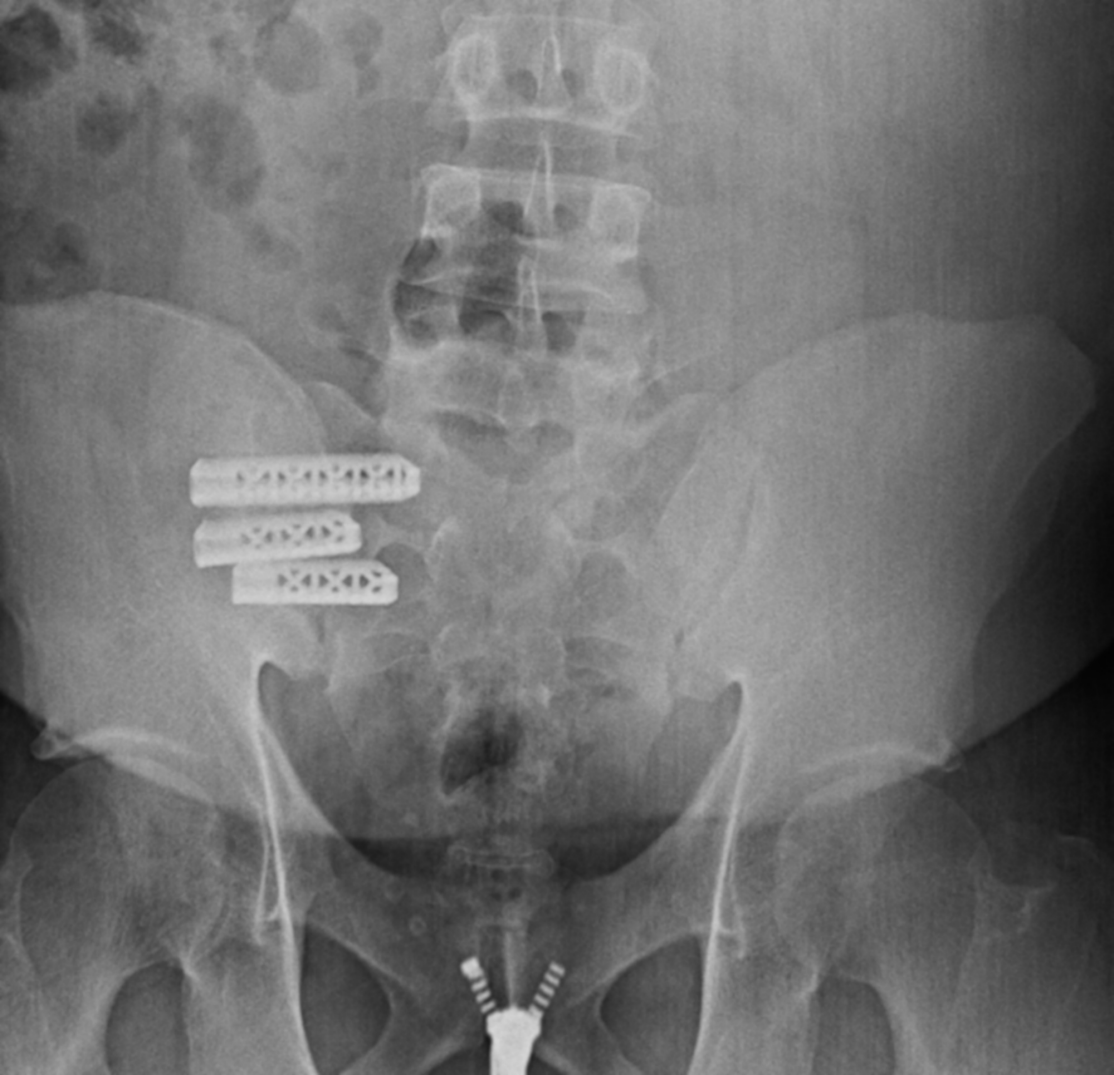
Six-month anteroposterior X-ray displaying the correct placement of implants
